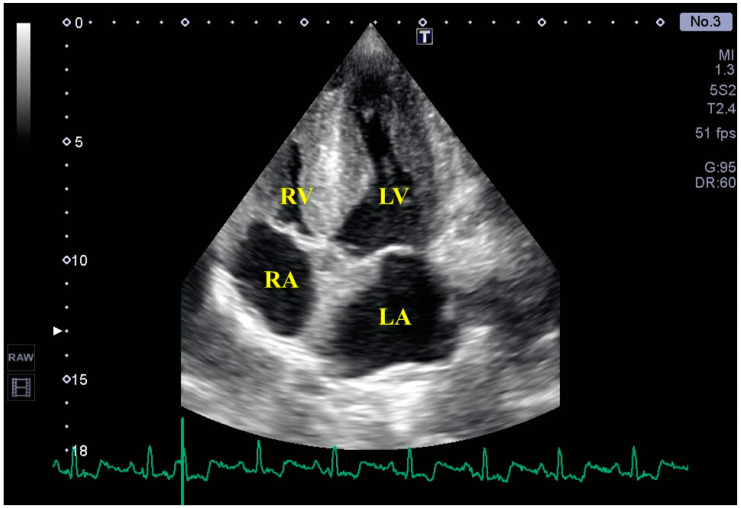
Routine two-dimensional echocardiographic image from the apical 4-chamber view of a patient with cardiac amyloidosis with biventricular hypertrophy. Abbreviations. LA = left atrium, LV = left ventricle, RA = right atrium, RV = right ventricle.
